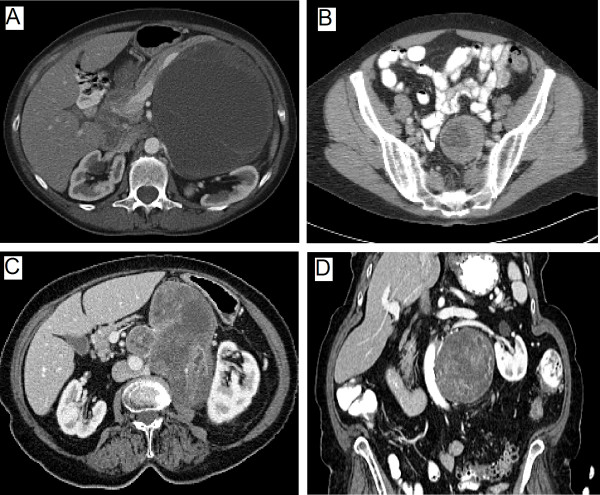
Computed tomography findings of three retroperitoneal ancient schwannomas. A. Large partly cystic and solid tumor displacing the pancreas and splenic vein anteriorly and compressing left kidney. Tumor concerning for a sarcoma or pancreatic neoplasm. B. Well defined tumor extending from the retroperitoneum into left pelvis adjacent to sigmoid colon. Region of vascular enhancement around tumor periphery can clearly be seen. C&D. Large heterogeneous enhancing mass adjacent to the aorta and left kidney shown in transverse and sagittal sections. Partial encasement of the aorta and left renal artery is demonstrated concerning for a malignancy.
